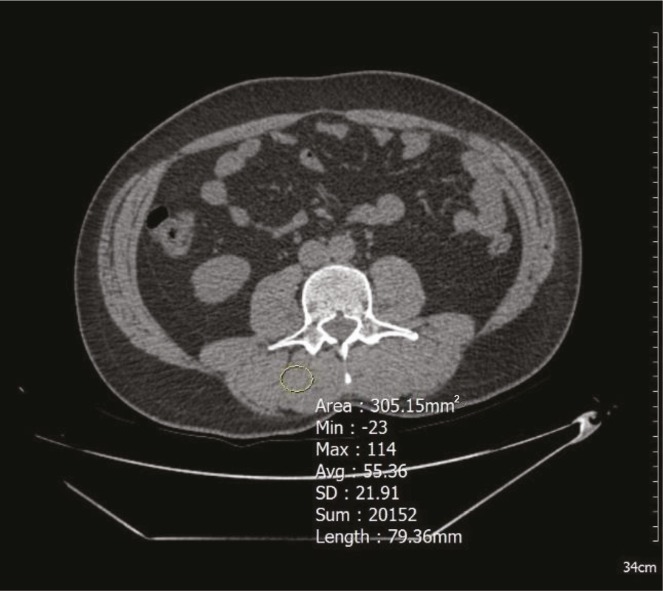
Muscle atrophy is evaluated visually using ROI on the CT image; according to visual evaluation, Grade 0 (normal), and muscle density measurement is 55 HU, which is recorded as normal muscle mass.
